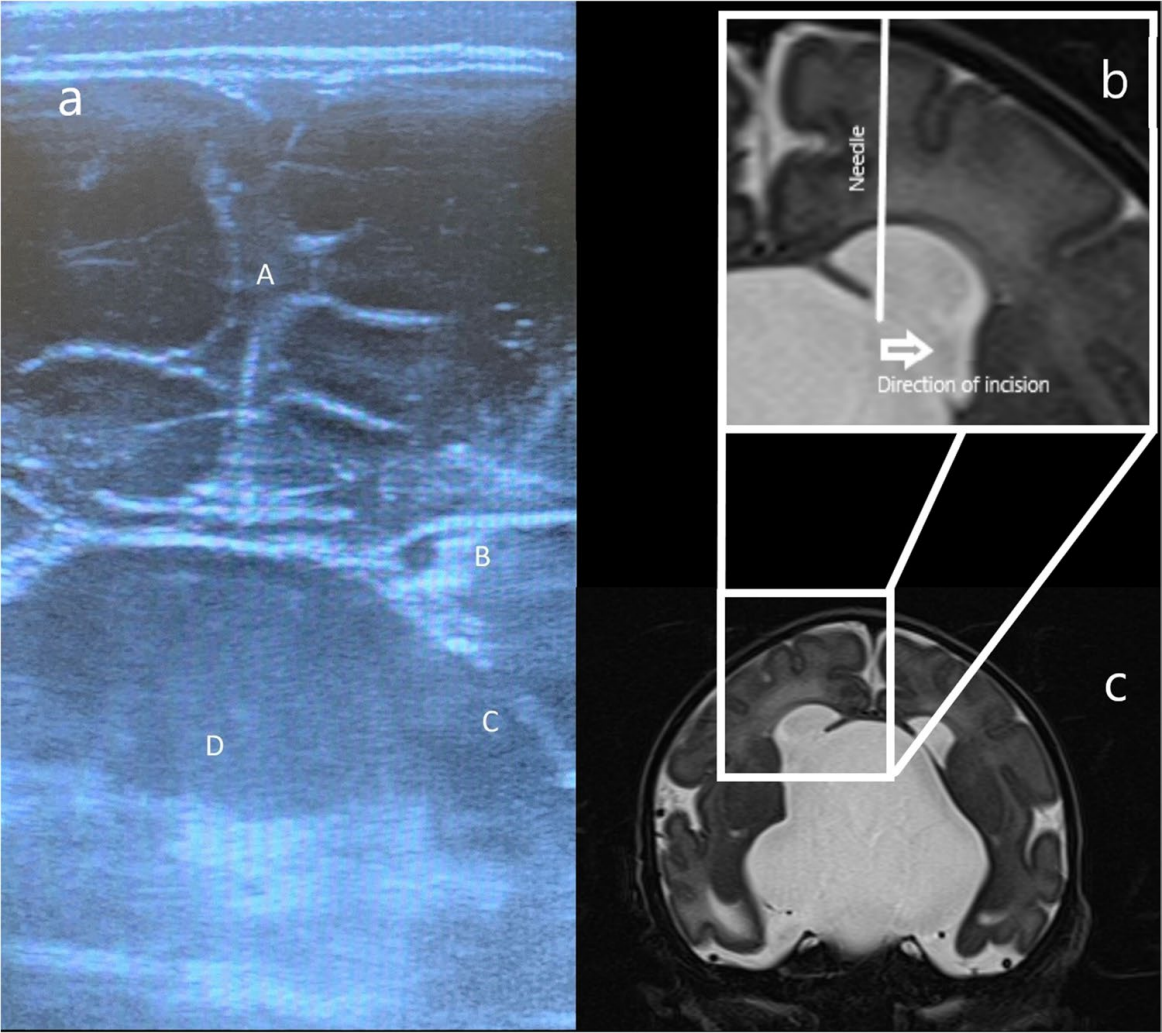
a Coronal ultrasound at level of foramen of Monro demonstrating needle point just traversing medial margin of arachnoid cyst as it presents into lateral ventricle. A; Falx cerebri, B; Needle tip, C; Cyst wall presenting via foramen of Monro, D; arachnoid cyst. b Magnified and flipped view of right lateral ventricle with needle path and trajectory of incision marked. c: coronal T2 weighted MRI scan through level of foramen of Monro
